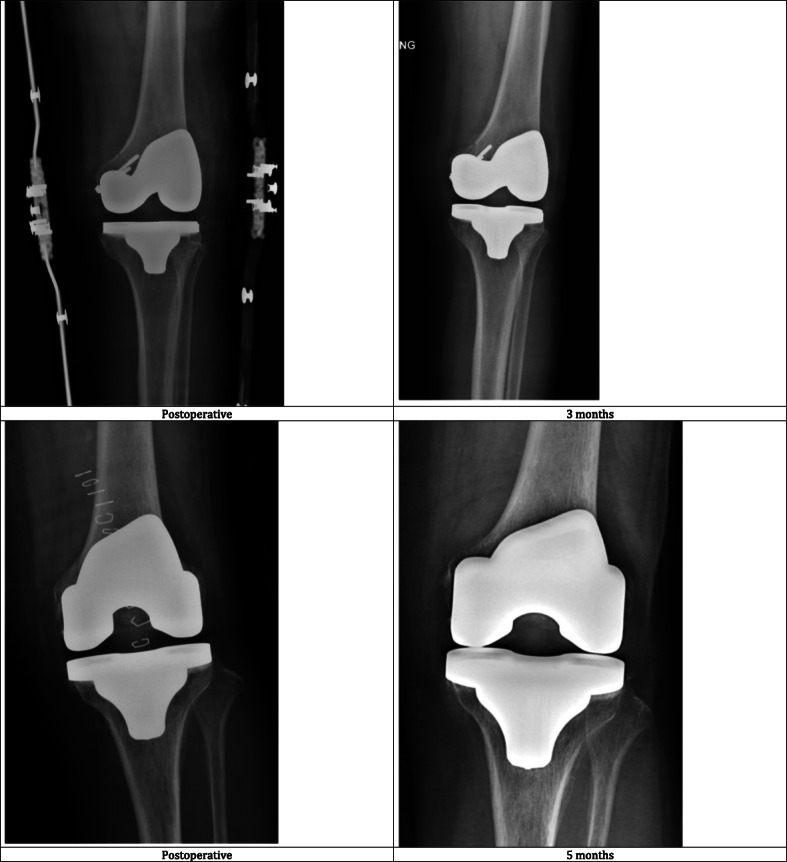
Postoperative radiographs of two patients who suffered from intraoperative femoral fracture
